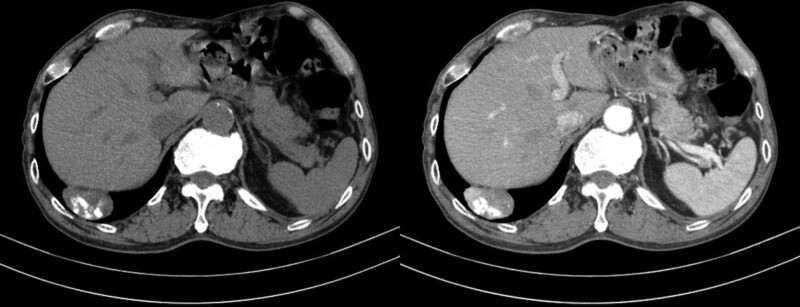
Initial computed tomography (CT) scan of the mass. Axial pre- and post-contrast mediastinal setting CT images (slice thickness, 3 mm) show a 45-mm, well-defined mass at the right lower lobe, abutting the diaphragm. The nodule has well-defined coarse calcifications and heterogeneous enhancement.
